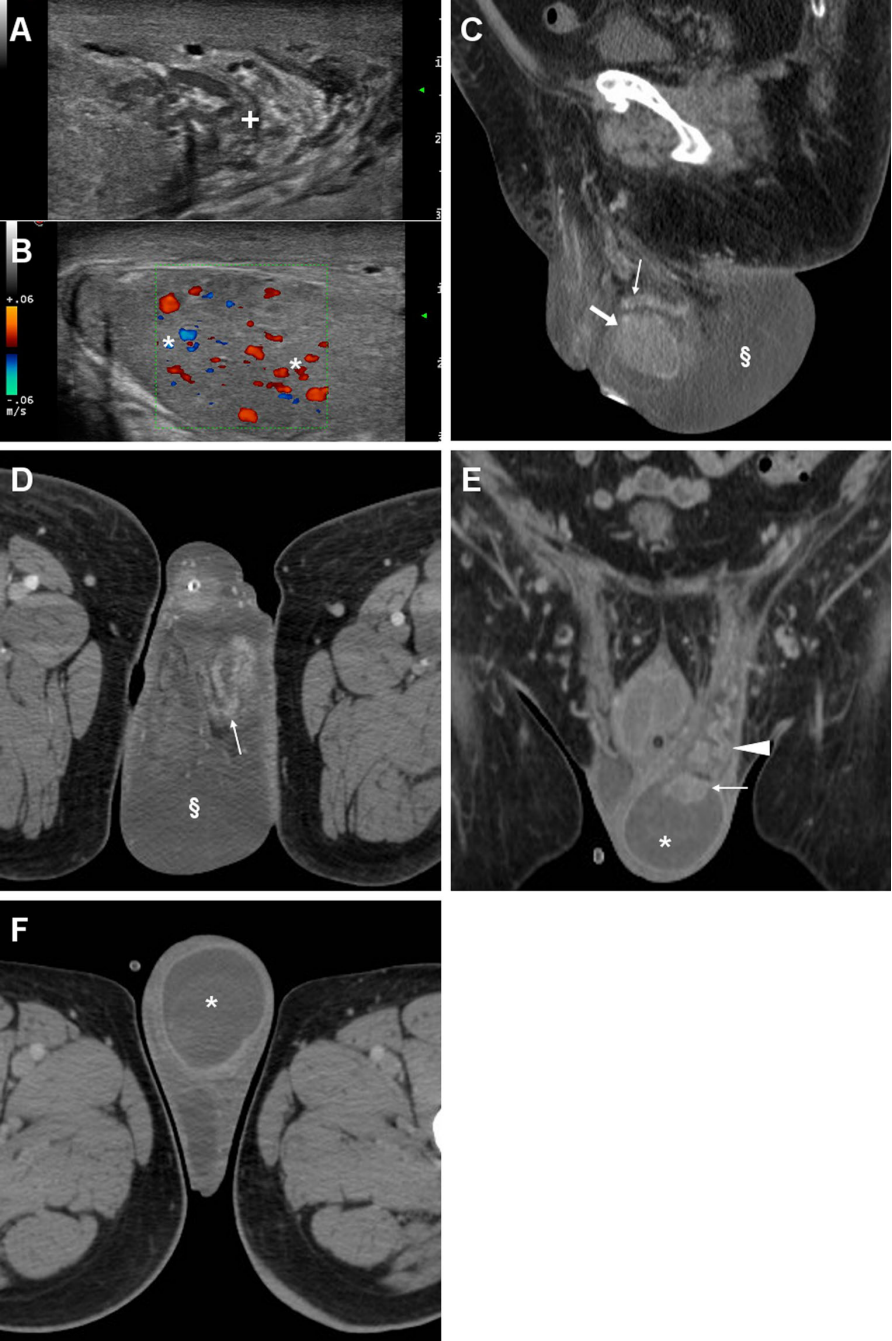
Surgically confirmed epididymo-orchitis with pyocele in a 72-year-old diabetic man with haematuria and enlarged left scrotum, history of transurethral resection of bladder carcinoma and bladder neck stricture treated by long-term catheterisation. Ultrasound revealed ipsilateral enlarged inhomogeneous epididymal head (+ in a) and hypervascularised testis (* in b). After unsuccessful antibiotic therapy, contrast-enhanced CT (c, d) showed hyperaemic left epididymis (*thin arrows*) and testis (*arrows*) compared to contralateral structures, and development of posterior scrotal collection (§). Another surgically proven case of testicular abscess and necrosis in a 59-year-old man with epididymo-orchitis unresponsive to medical therapy: post-contrast CT (e, f) revealed vascular engorgement along the left spermatic cord (*arrowhead*), faintly enhanced epididymal head (*thin arrow* in e), and ipsilateral scrotum occupied by fluid-like collection (*) with thin peripheral enhancing rim
